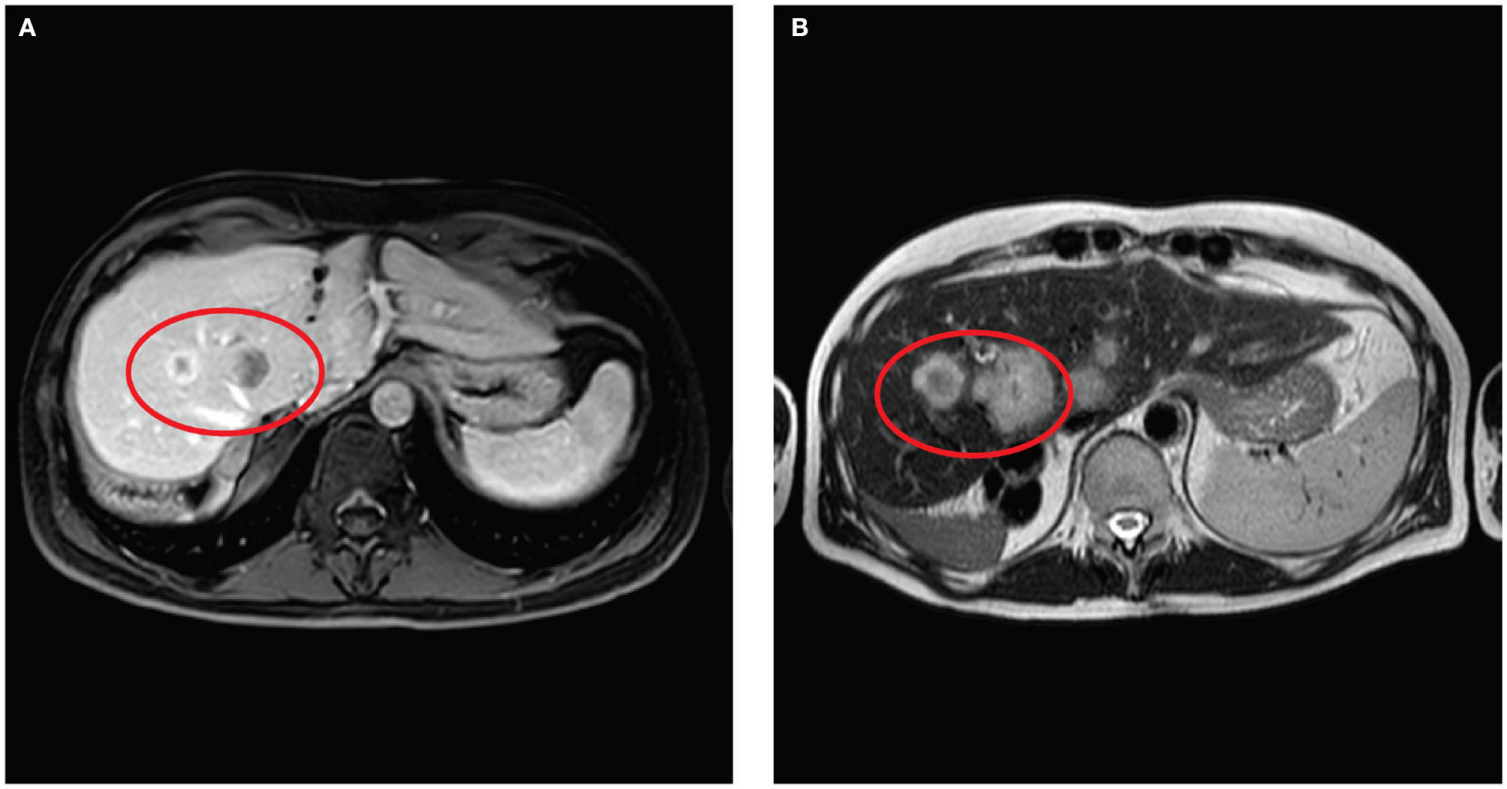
(A) Magnetic resonance post-contrast (gadolinium enhanced) axial MRI performed 6 months before surgery show low peripheral enhancement. (B) Axial MR T2 WI, performed 1 month before surgery, show slightly hyperintense lesions visible in the red circle referable to colangiocarcinoma recurrence, located at the hepatic hilum, quickly grow in size.
